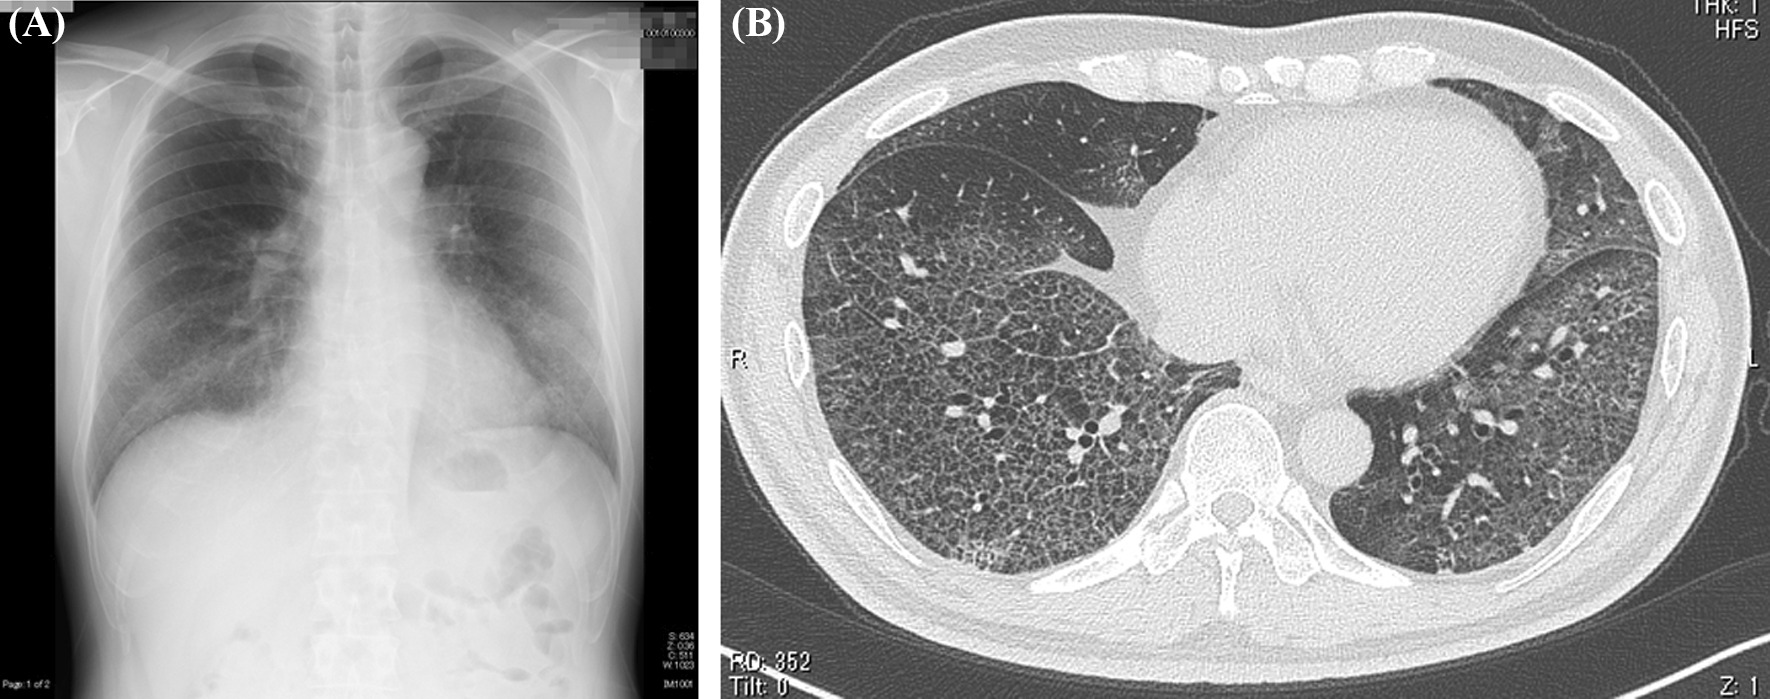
A Plain radiograph of the chest (day T + 8). Note the extensive frost shadows in the upper and lower lung fields with bilateral peripheral predominance. B Computed tomography image of the chest (day T + 8). Note the broad range of ground-glass opacity with interlobular septal thickening and bilateral peripheral dorsal base predominance.* T* Day patient became febrile (38 °C). Figure is taken from Kashiwabara *et al.* [5], with permission
